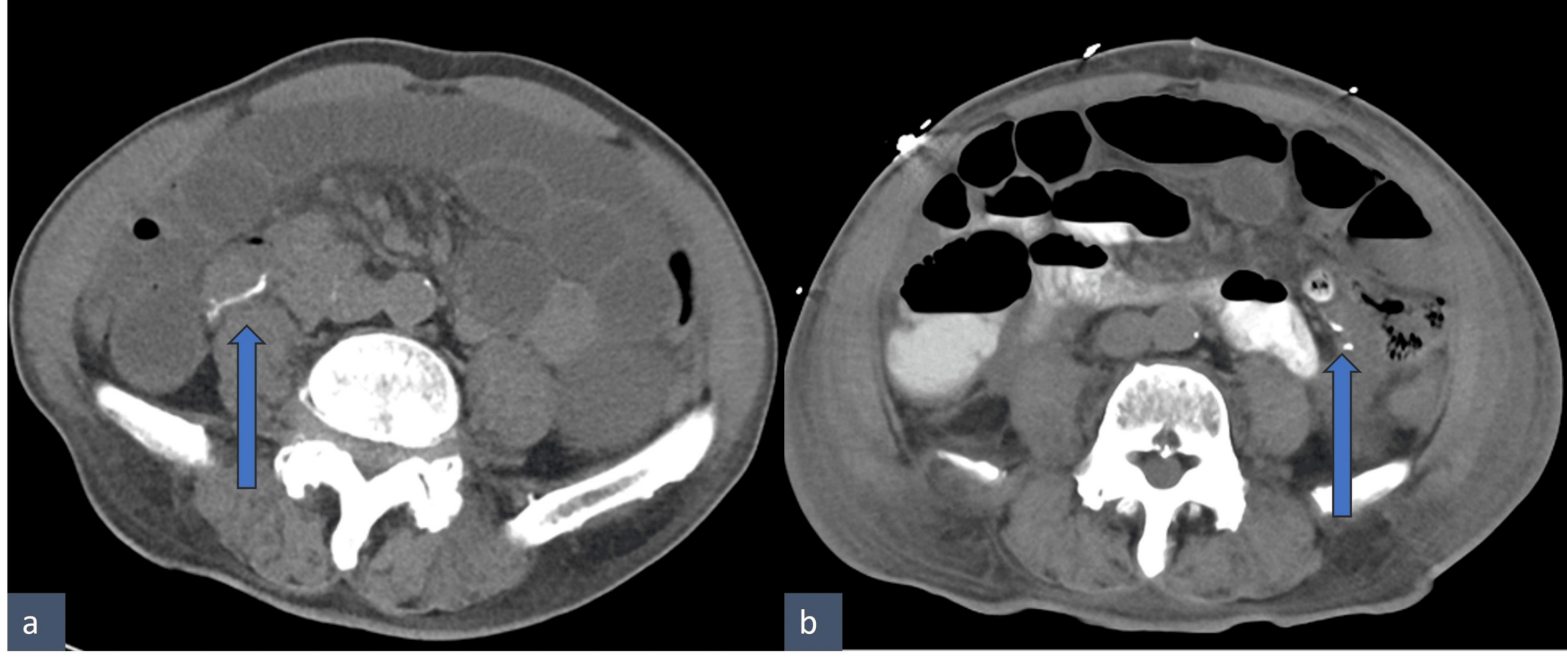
Axial pre- (a) and post-surgery (b) CT abdomen Cecum, marked by blue arrows, is in the medial location, inferior to the duodenum pre-surgery and lateral on the left of the abdomen post-surgery. CT: computed tomography
